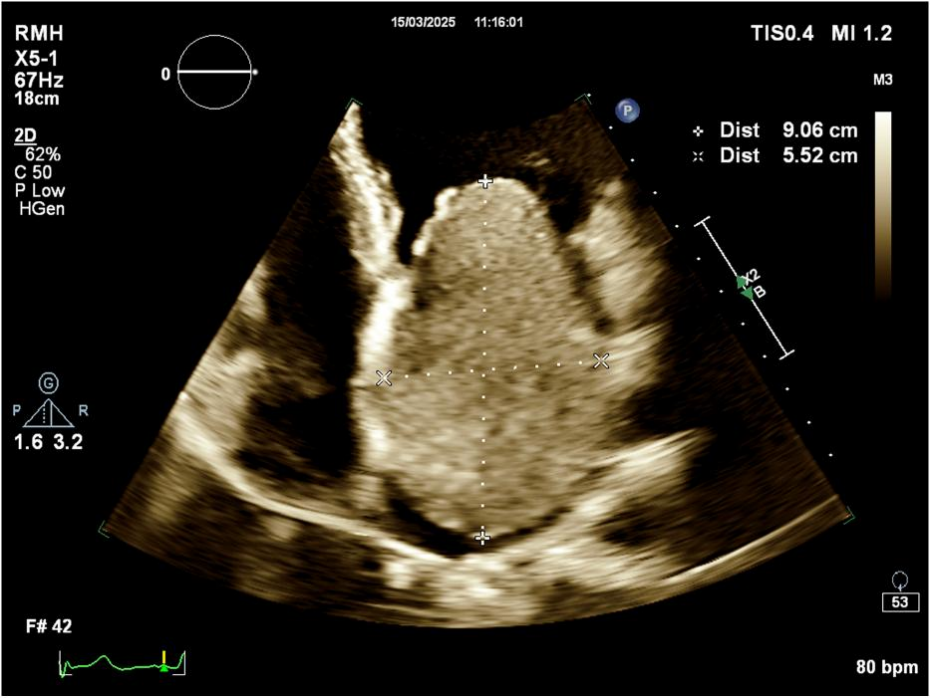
Transthoracic echocardiogram demonstrating the massive left atrial mass which measures 9.1 × 5.5 cm.
